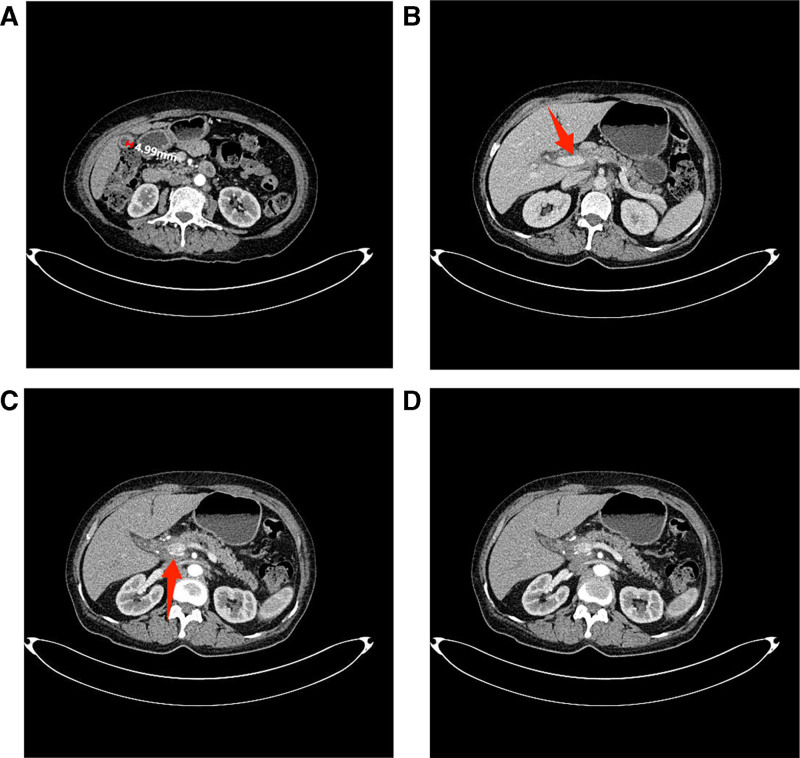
Post-chemotherapy CT findings. (A) The thickness of the gallbladder fundal wall measures approximately 4.99 mm. (B) Marked improvement in portal vein invasion (marked by the red arrow). (C) Marked improvement in hepatic artery invasion (marked by the red arrow). (D) Multiple enlarged lymph nodes in the porta hepatis and retroperitoneum have significantly regressed compared to the pre-chemotherapy scan.
